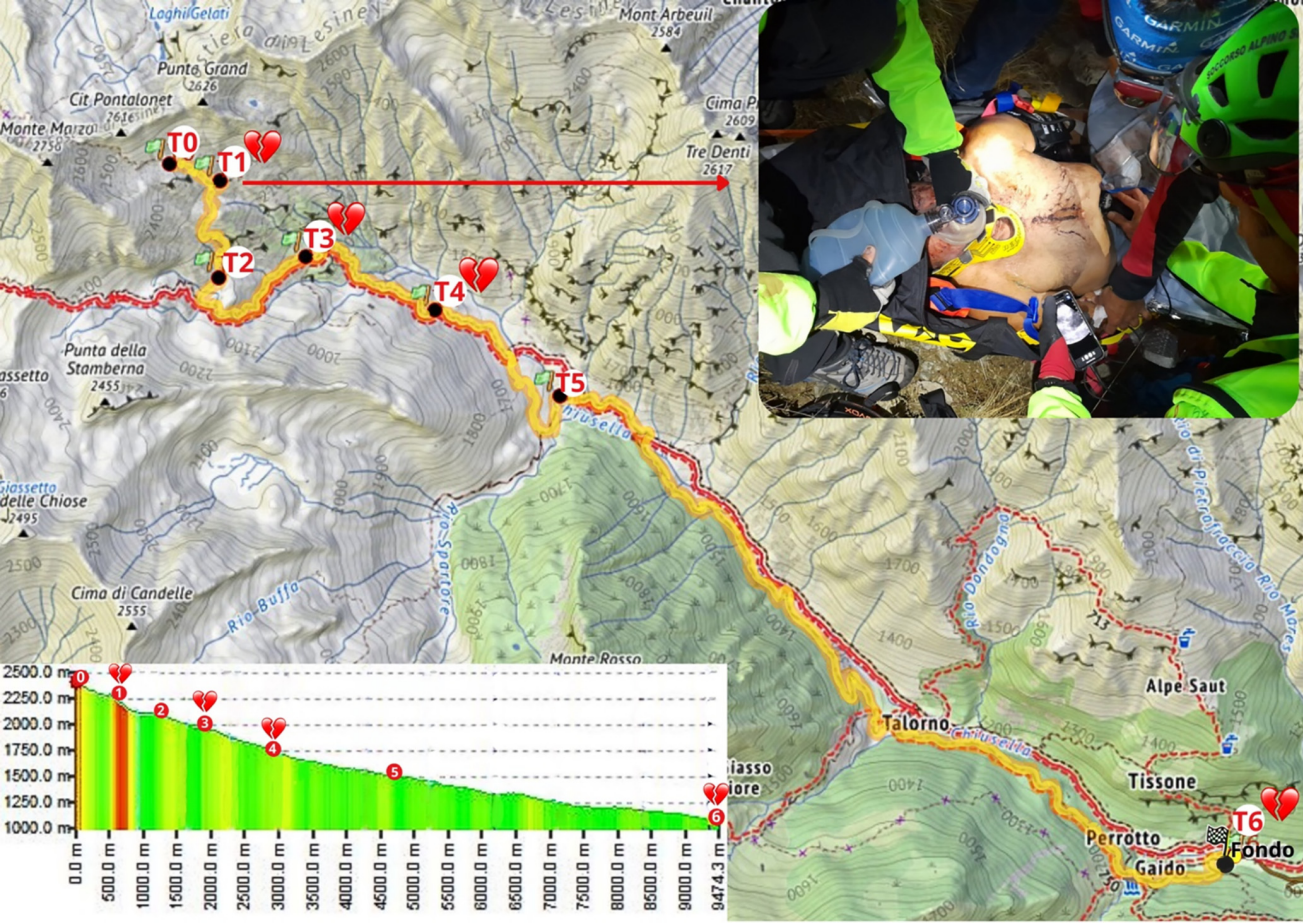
Map showing the timeline and location of the different time points (T0–T6) of the rescue operation. The heart icons indicate the four time points at which cardiac arrest (CA) occurred. T0 (2412 m above sea level): rescue team dispatched to the target point; T1 (2318 m): first cardiac arrest during a verticalization maneuver; T2 (2104 m): missed rendezvous with the helicopter, the patient was conscious; T3 (1956 m): second cardiac arrest; T4 (1756 m): third cardiac arrest, intramuscular adrenaline (0.5 mg) administration was started every 20–30 min; T5 (1567 m): patient transferred to a dirt off-road rescue vehicle; T6 (1072 m): rendezvous with ambulance, fourth cardiac arrest. The lower left box shows altitude above sea level (y-axis) and the distance in meters (x-axis) from the beginning of the rescue (T0) to the point where the patient was loaded into the ambulance (T6). The upper right box shows a photo of the patient undergoing an E-FAST (Extended Focused Assessment with Sonography for Trauma) examination.
